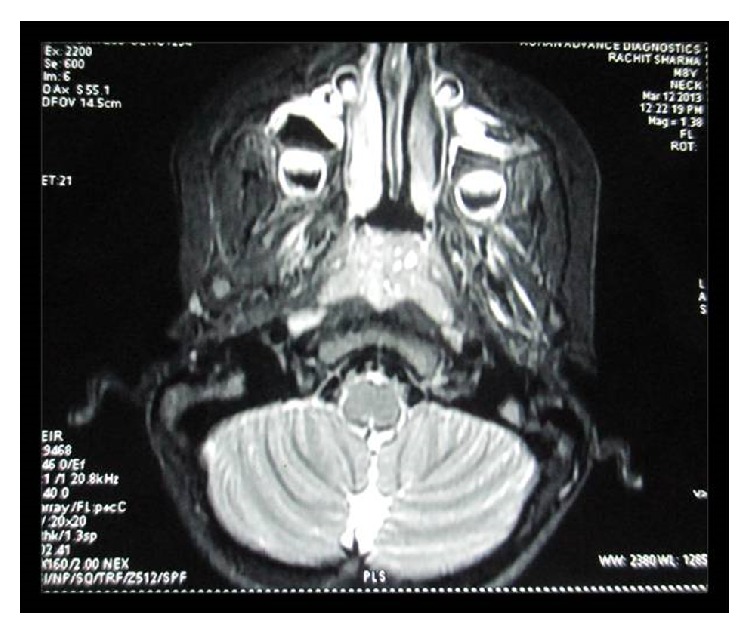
MRI showing aplasia of lacrimal and salivary glands.
